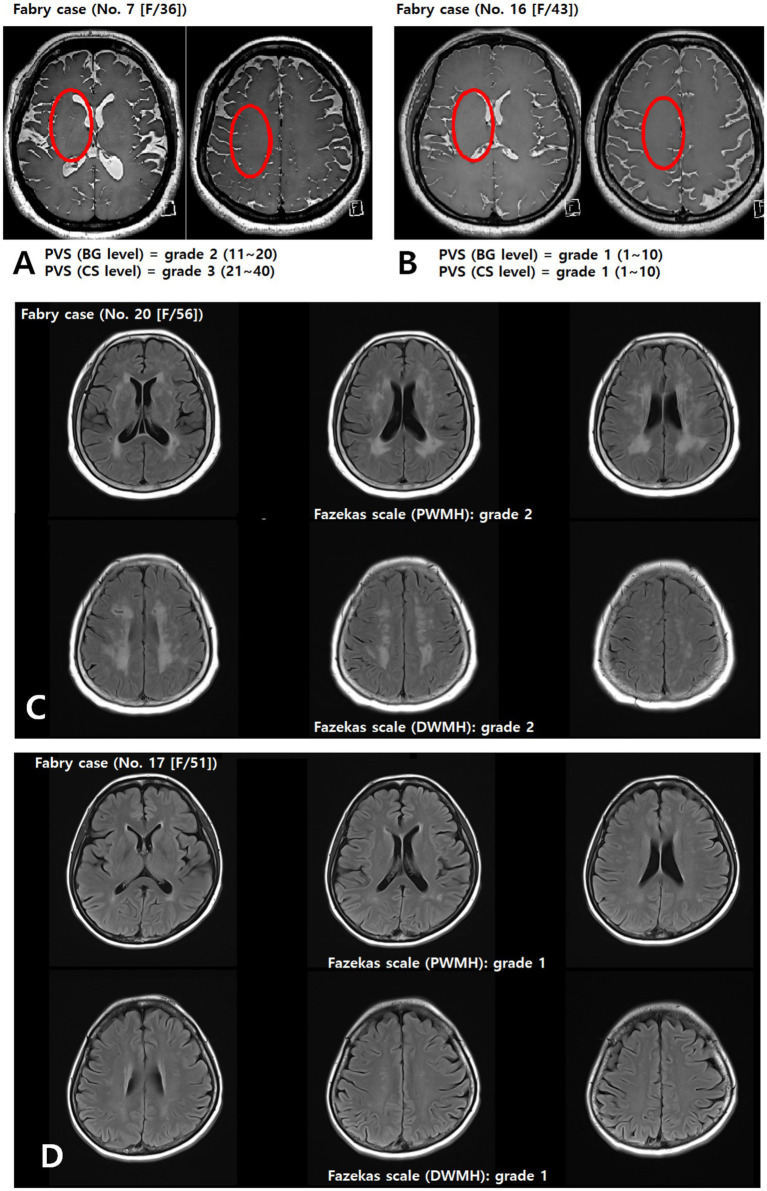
Assessment of the PVS (A,B) and Fazekas (C,D) scales. Representative axial T2-weighted image of a patient with FD showing multiple enlarged perivascular spaces (red circle) in the basal ganglia and centrum semiovale (A). BG, basal ganglia; CS, centrum semiovale; DWMH, deep white matter hyperintensity; PVS, perivascular space; PWMH, periventricular hyperintensity.
